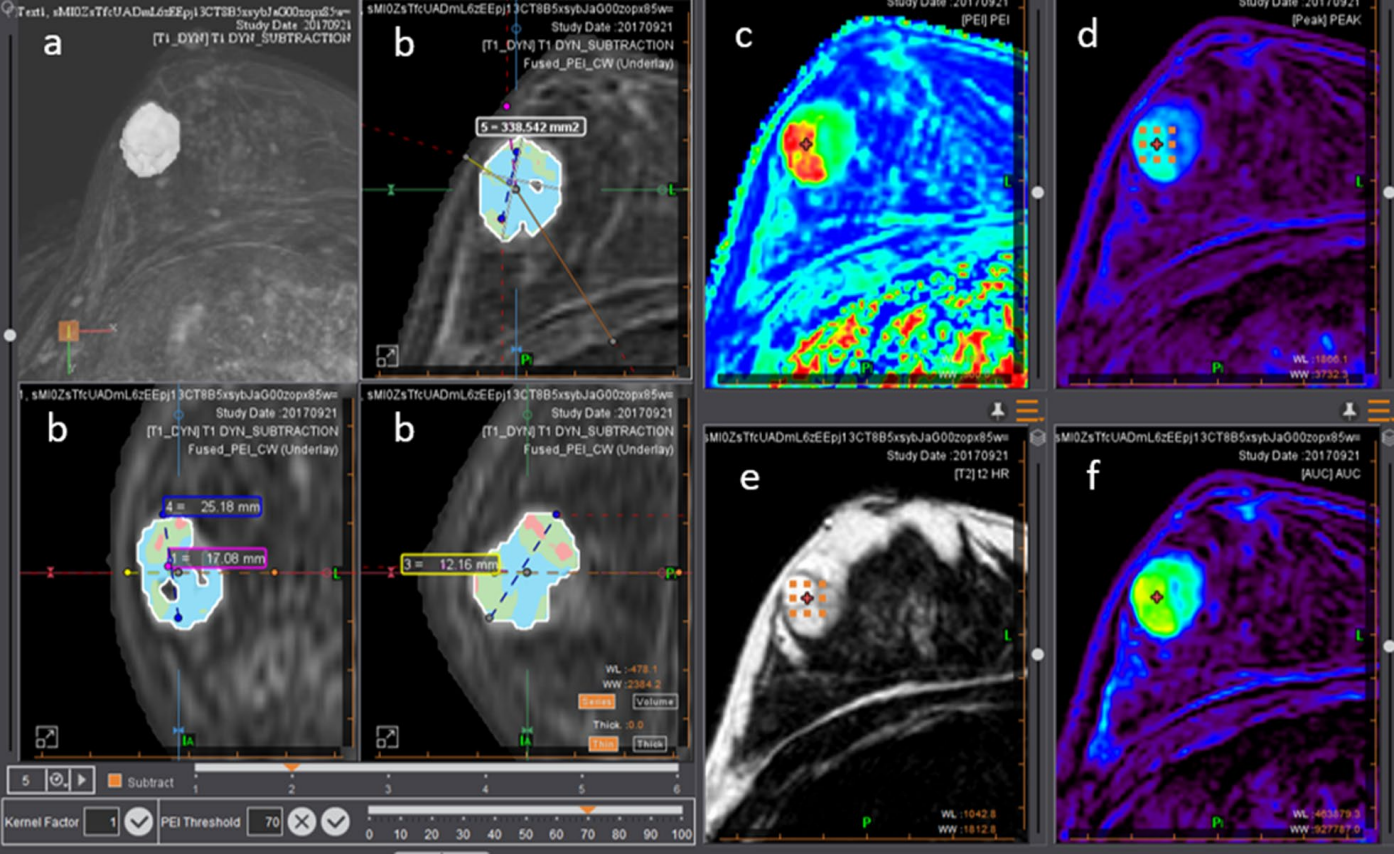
Example of a fibroadenoma at the junction of the external quadrants of the right breast on the breastscape^®^ segmentation software: a Dynamic contrast enhancement subtraction with MIP reconstruction; b Dynamic contrast enhancement sequence merged with the PEI map in the axial, coronal, and sagittal planes; c PEI map; d PEAK map; e T2-weighted sequence in axial view; f AUC map
